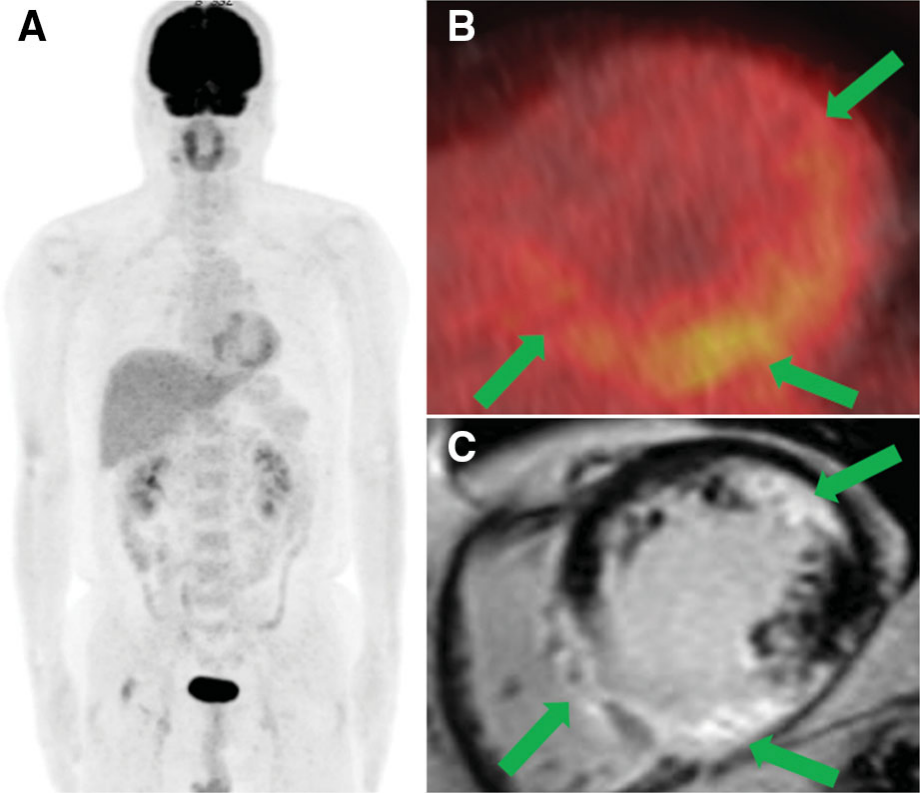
Images of a representative case of isolated cardiac sarcoidosis. A 53-year-old man with sustained ventricular tachycardia. Echocardiography detected left ventricular (LV) contractile dysfunction with an ejection fraction of 49% and thinning of the LV wall (No.1, Online Resource 2). The maximum intensity projection image of positron emission tomography (PET) showed no abnormal ^18^F‐fluorodeoxyglucose (FDG) uptake other than the heart (A). The PET/CT fusion image revealed abnormal FDG uptake of focal-on-diffuse pattern in the left ventricular myocardium (B). Cardiovascular magnetic resonance showed late gadolinium enhancement, consistent with abnormal FDG uptake (C). There was no evidence of extracardiac sarcoidosis, and the patient was diagnosed with isolated cardiac sarcoidosis since he met the five major criteria
